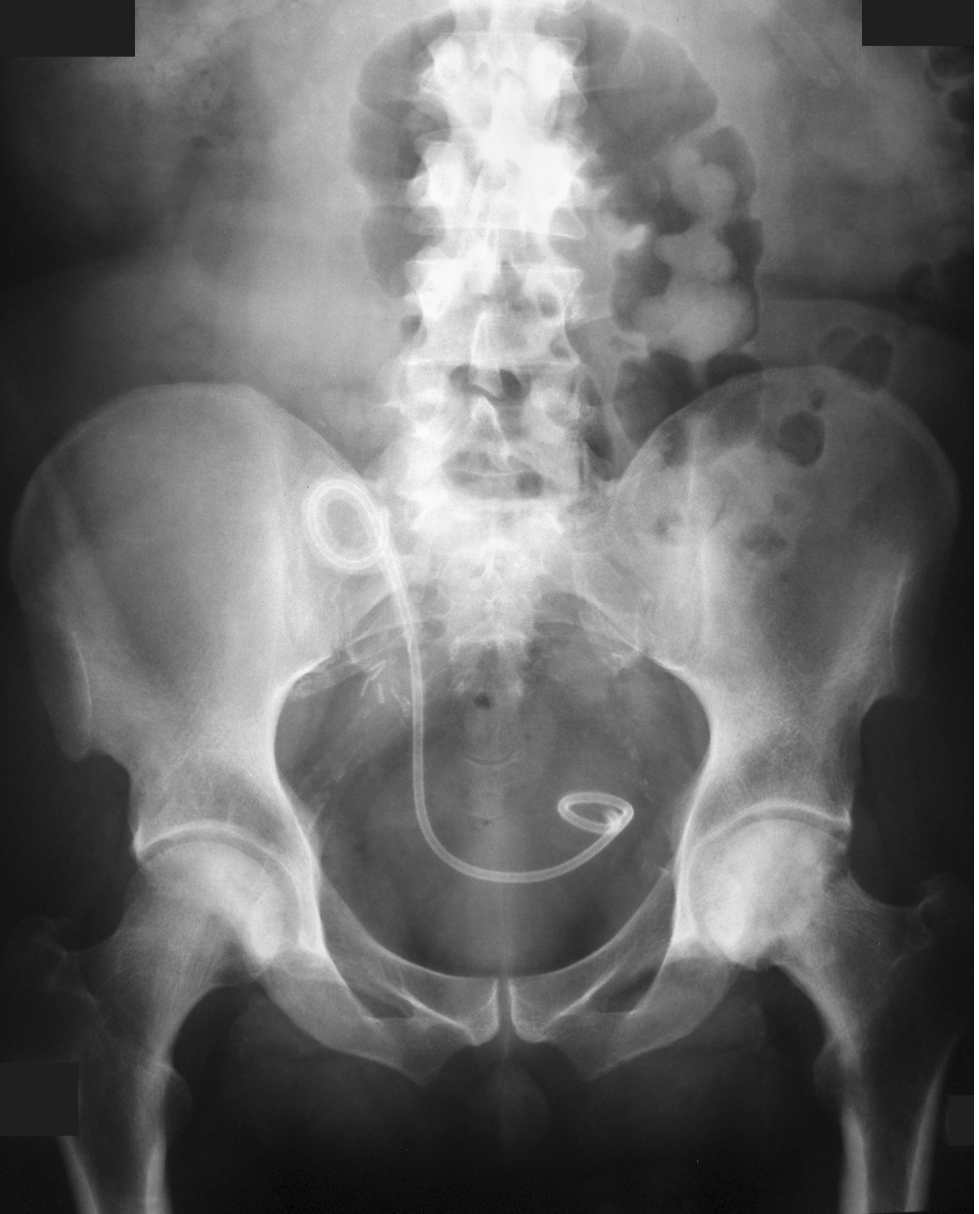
Plain film demonstrating encrusted stent.
